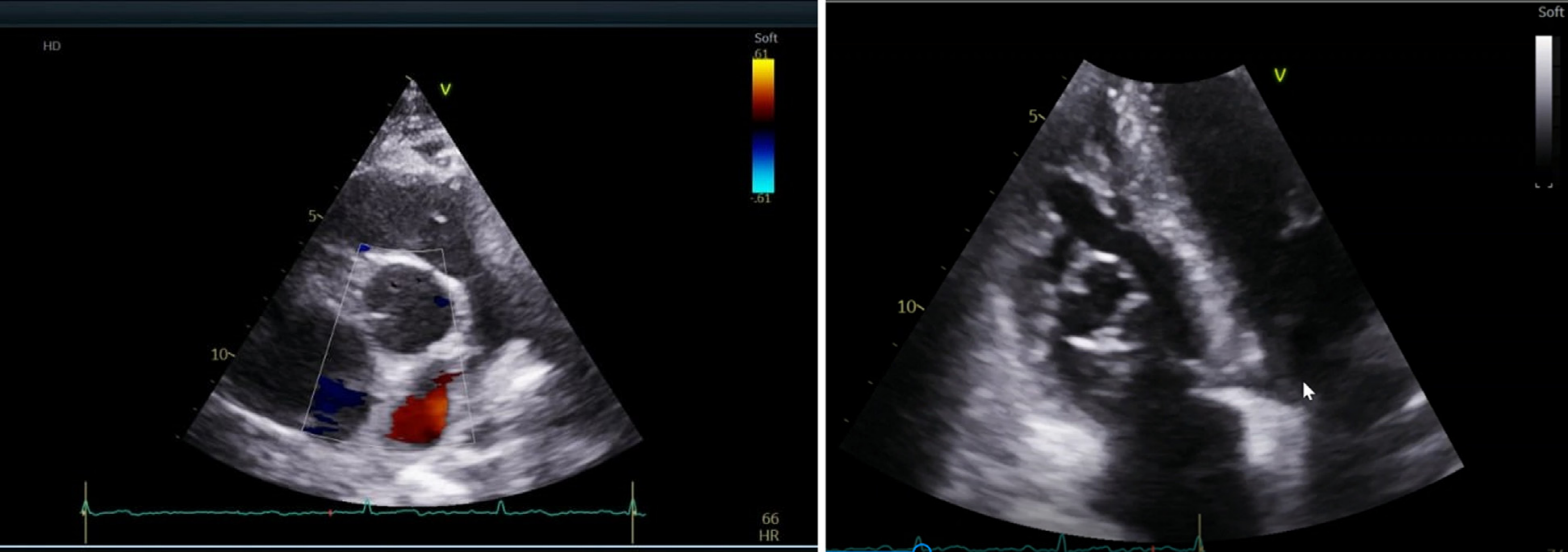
TTE Revealing RV Cystic Masses Attached to the RV Free Wall (A and B) TTE (aortic valve in short-axis parasternal view) showing cystic masses attached to the RV free wall. The left ventricle appeared normal with preserved systolic and diastolic function; the ejection fraction was 60%. A large mass measuring 2.4 × 2.1 cm was visualized in the RV, attached to the RV free wall just below the lateral tricuspid valve annulus. A mobile component was noted on the mass surface. Differential diagnoses included secondary cardiac masses. No significant valvular abnormalities were observed. RV = right ventricle; TTE = transthoracic echocardiography.
